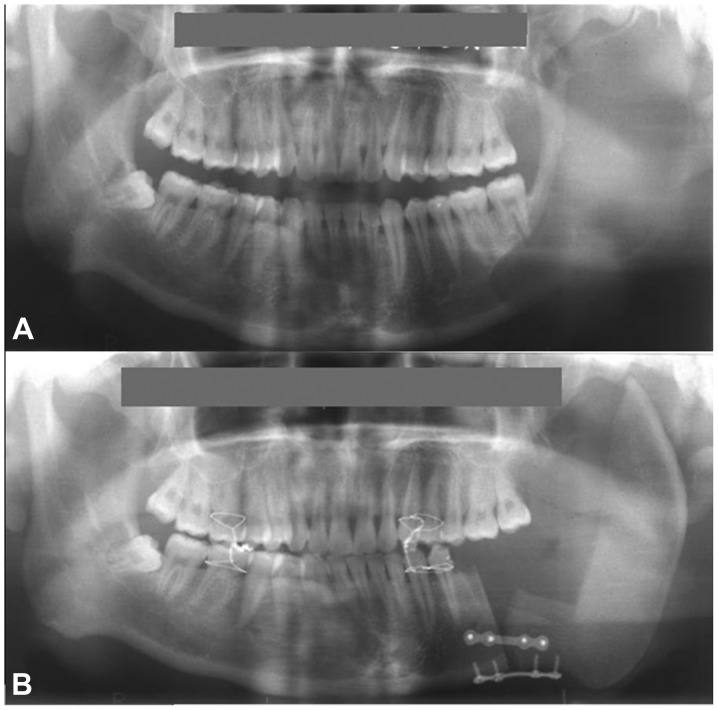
Panoramic radiographs showing (A) the unilocular radiolucent lesion of the left mandible and (B) reconstruction following the first surgery.
